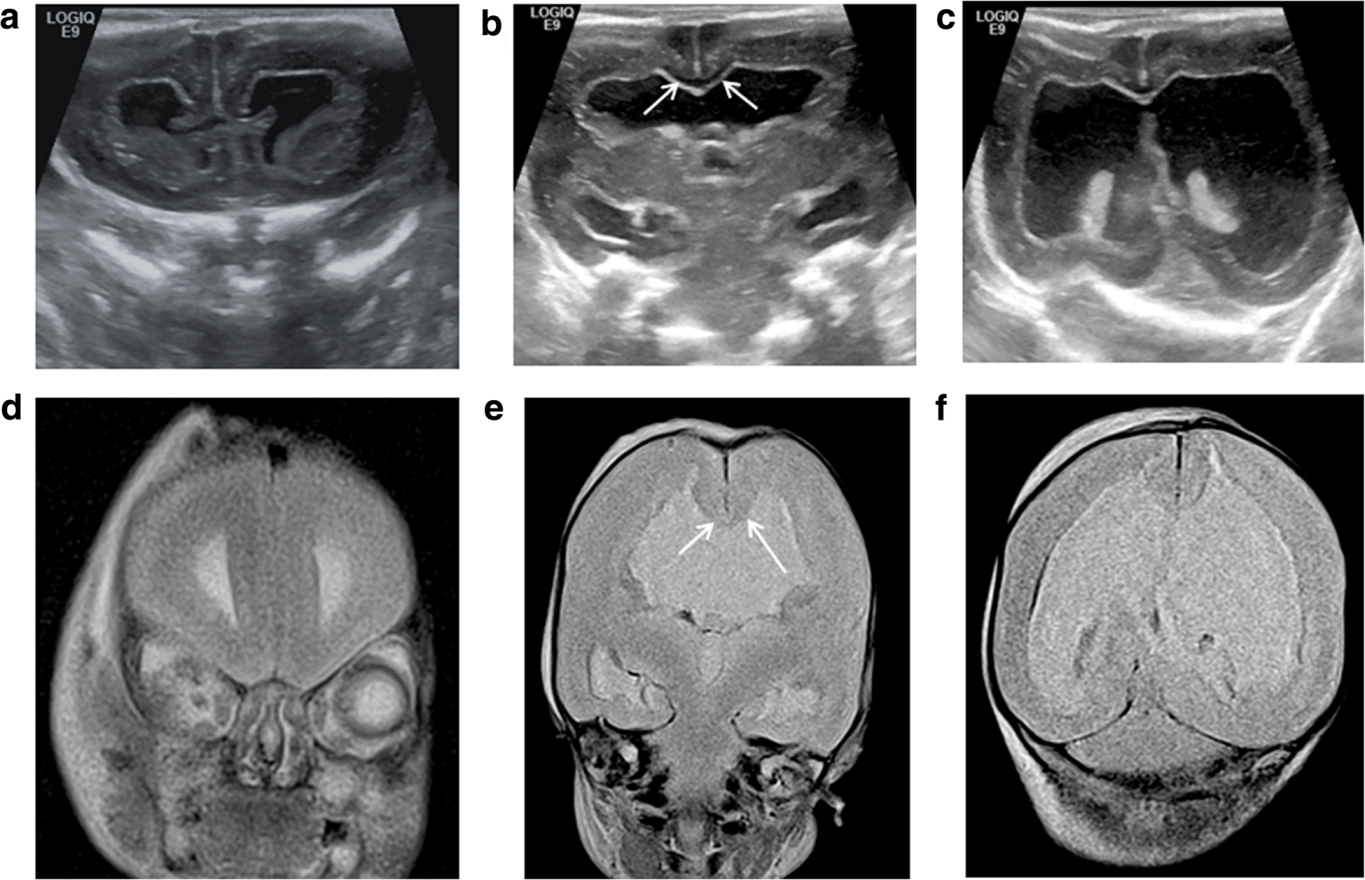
Post-mortem ultrasound images of the brain, in coronal section (top row), with matched T2-weighted post-mortem MRI images (bottom row) performed on the same day, in a foetus at 21 weeks gestation, 9 days after death. These have been obtained through the frontal lobe (a, d), at the level of the Foramen of Monro (b, e) and through the posterior horns of the lateral ventricles (c, f). The pregnancy was terminated for suspected foetal ventriculomegaly and an absent corpus callosum. From the post-mortem ultrasound, the ventriculomegaly was well depicted before the MRI was performed, and a corpus callosum was in fact present (white arrows)
